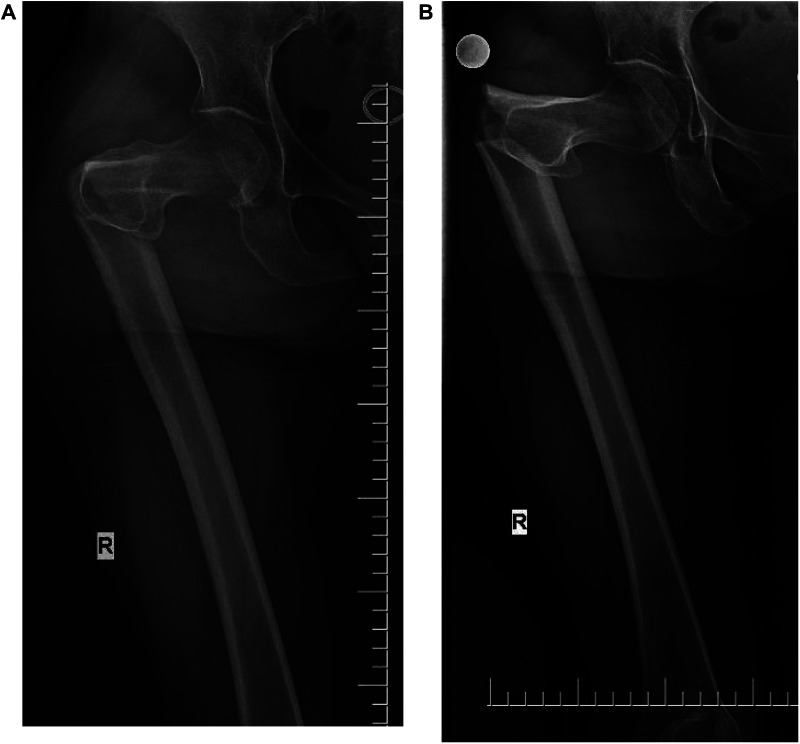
(A) Anterior radiograph fracture with angular deformity. (B) The fracture was significantly displaced, with proximal posterior rotation.
